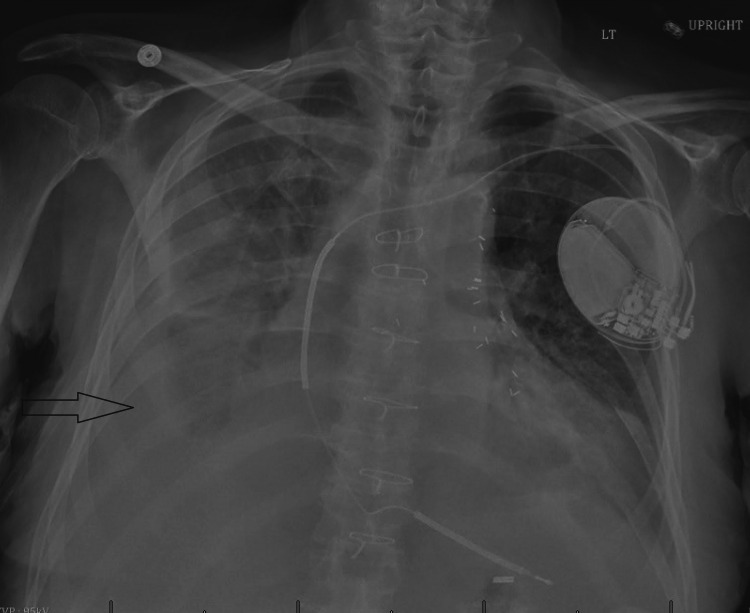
Chest X-ray showing right-sided pleural effusion (arrow)
